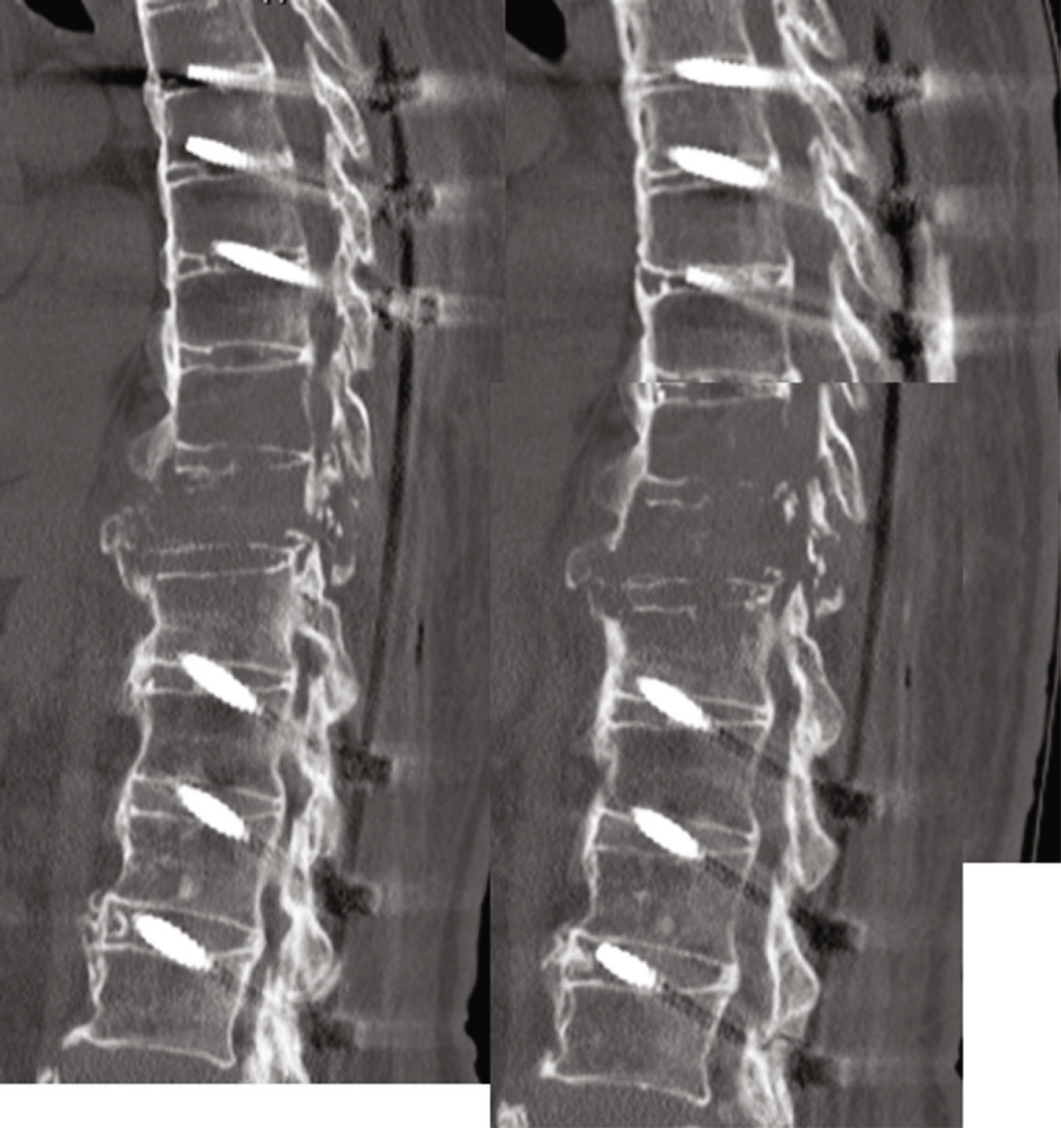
Postoperative CT showing appropriate implant placement and satisfactorily strong stabilization.
